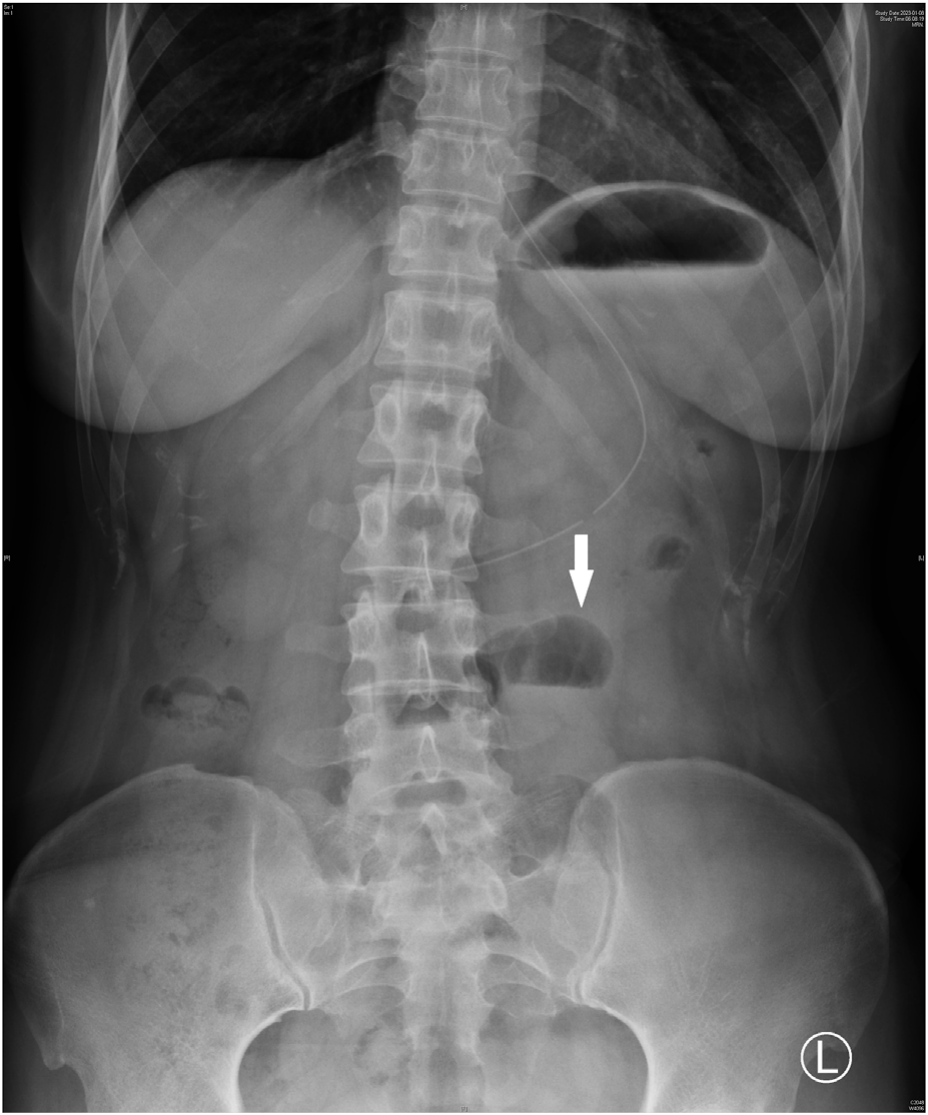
Abdominal X-ray imaging showed gas-liquid level in the mid-abdomen (white arrow).
